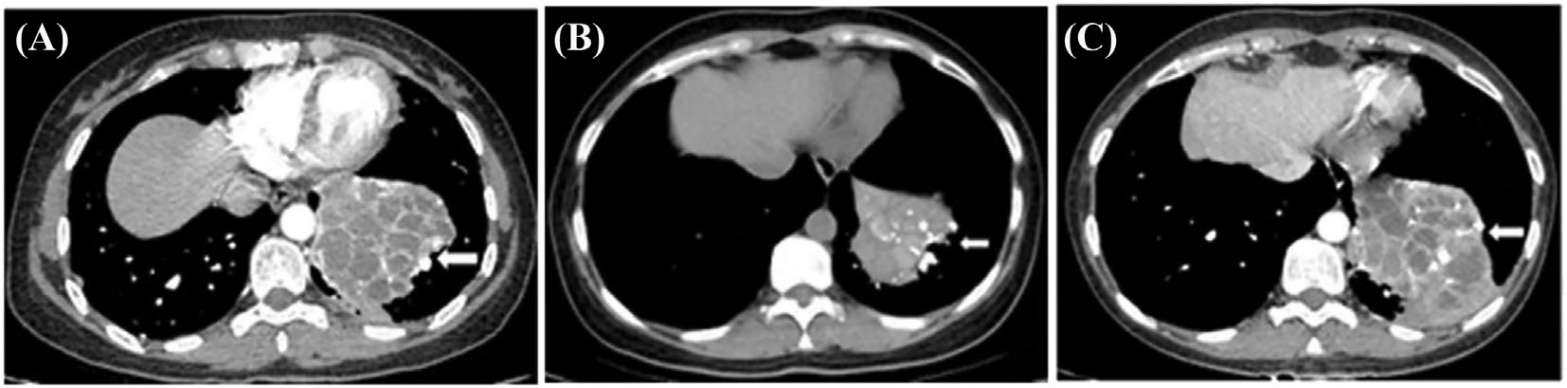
Computed tomography scan of chest, showing huge irregular solid mass with internal compartmentation in the lower lobe of the left lung: (A) before treatment with voriconazole, (B) after 6 months of treatment with voriconazole, and (C) after 3 weeks of discontinuation of treatment with voriconazole.
